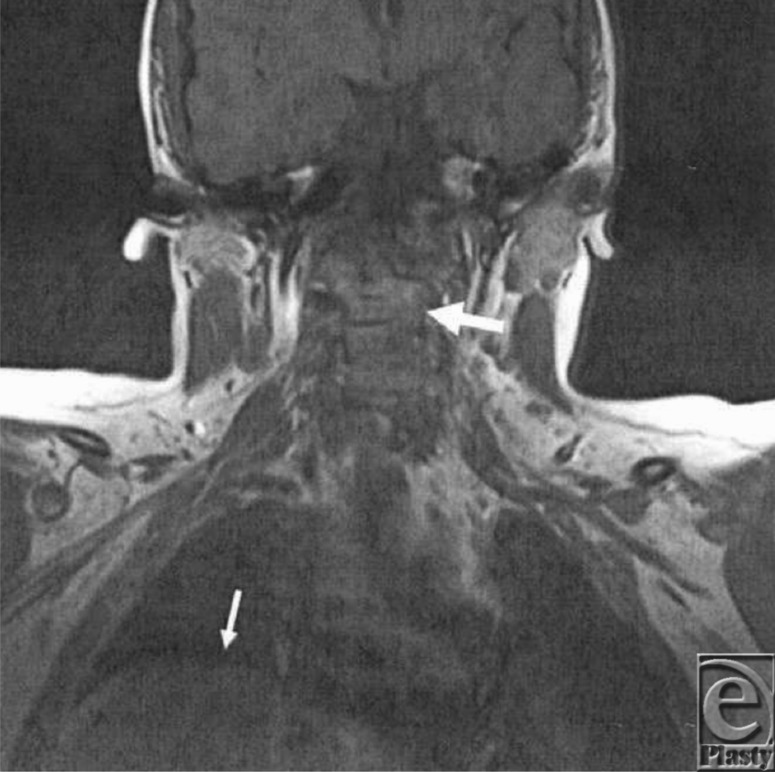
Coronal plane, T1-weighted sequence before surgery showing the fused cervical vertebrae (big arrow). We also noted elevation of the right hemidiaphragm (small arrow) indicative of unilateral diaphragmatic paralysis.
